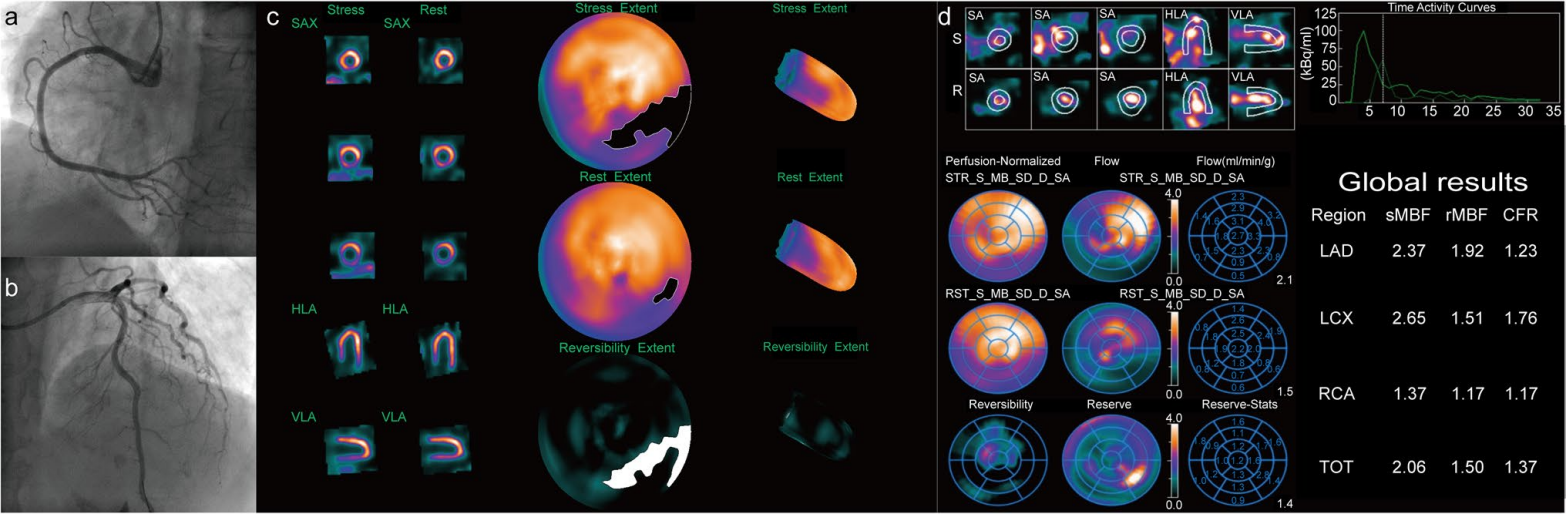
A 59-year-old male patient with hypertension and smoking history was referred for D-SPECT MBF quantification and ICA due to recurrent chest pain. a ICA indicates 30% stenosis of the right coronary artery (RCA), thrombolysis in myocardial infarction (TIMI) flow grade 3. b ICA indicates 30%–40% stenosis of the left anterior descending (LAD) coronary artery, TIMI flow grade 3. c Myocardial perfusion imaging (MPI) suggests partial reversible myocardial ischemia in the left circumflex artery (LCX) and RCA dominate segment. QPS/QGS quantitative analysis results: summed stress score (SSS) = 7, summed rest score (SRS) = 2, summed difference score (SDS) = 5. d MBF quantification showed significant decrease of MBF and CFR both regional and global. After 24 months of follow-up, this patient emerged with a nonfatal myocardial infarction
